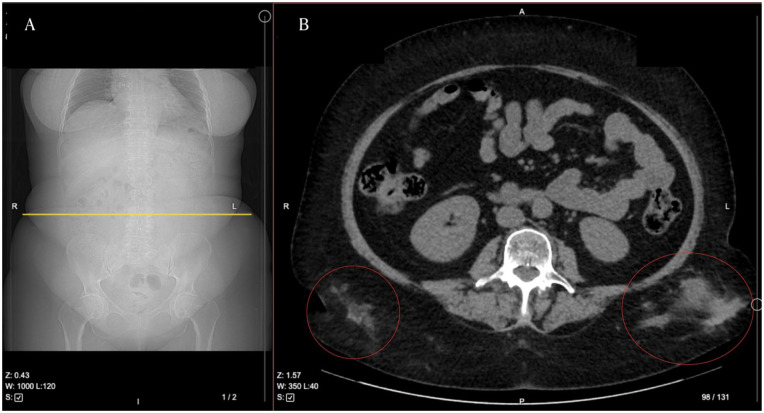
A section of whole-body CT. Yellow line (A) indicates the placement of the axial CT view depicted in (B). Red circles mark an abscesses in the gluteal region. R—right, L—left, A—anterior, P—posterior.
